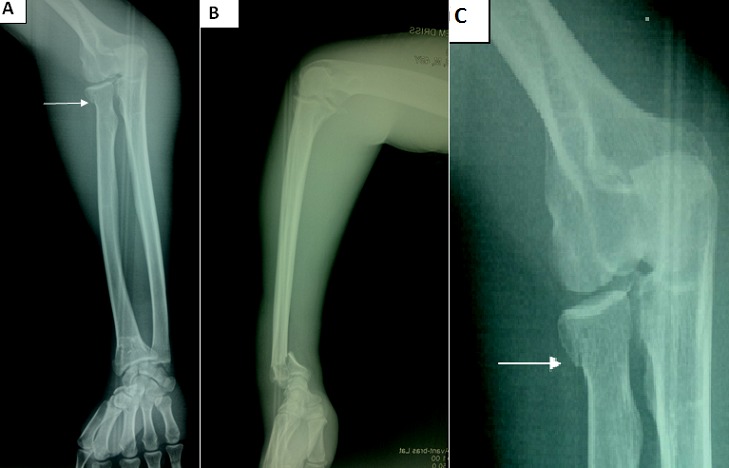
Preoperative radiograph of the right forearm, showing a combination of fractures of the distal and proximal ends of the radius (A, B); the undisplaced radial neck fracture (arrow) (C)
